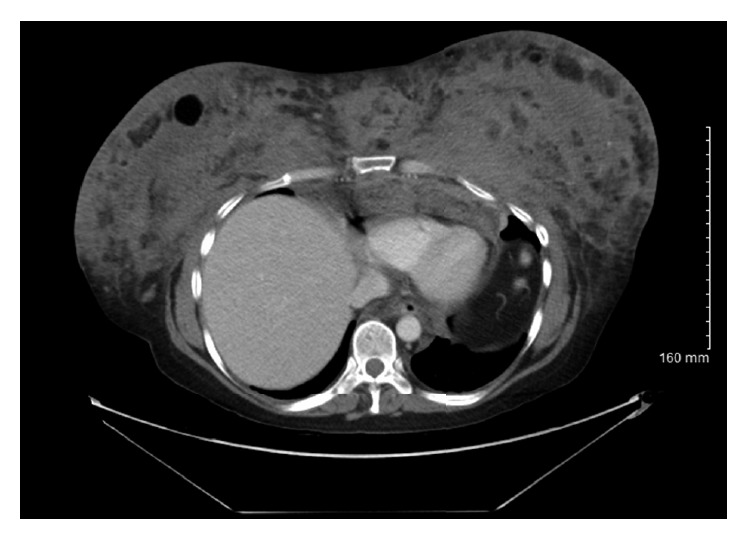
CT chest 2007: breast predominantly fibrous in composition with multiple well-circumscribed areas of fat throughout the breast parenchyma.
